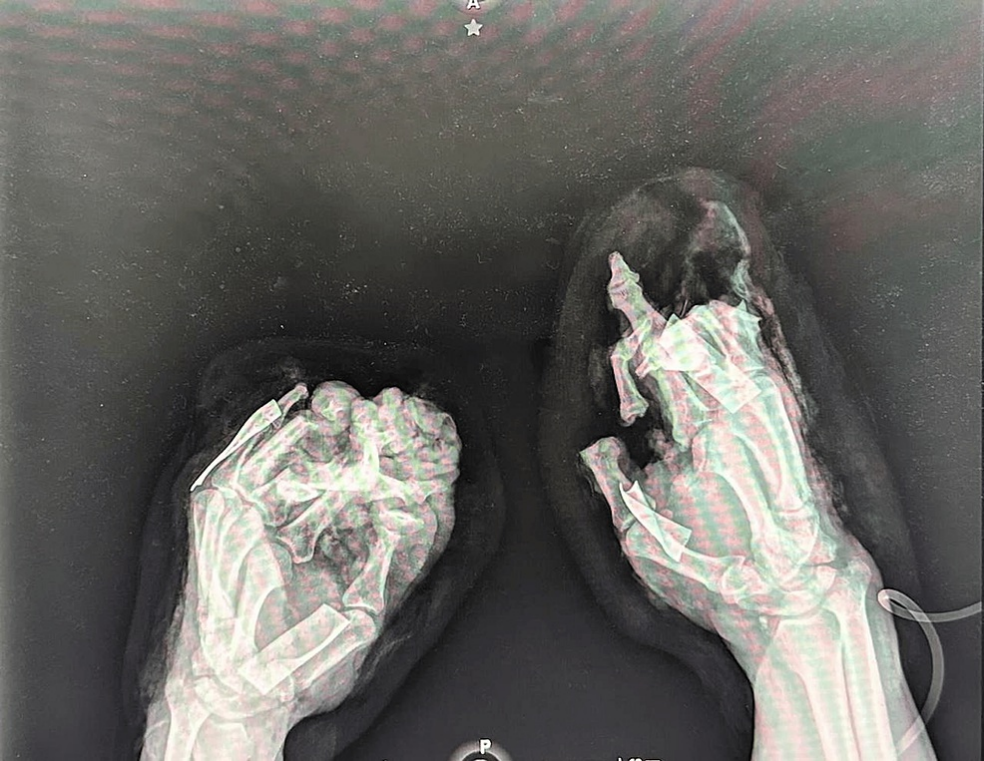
Preoperative X-ray, oblique projection of both hands. The radiographic image demonstrates the traumatic amputations of the left hand involving loss of proximal, middle, and distal phalanges of the third, fourth, and fifth fingers, and the distal phalanx of the first finger.
